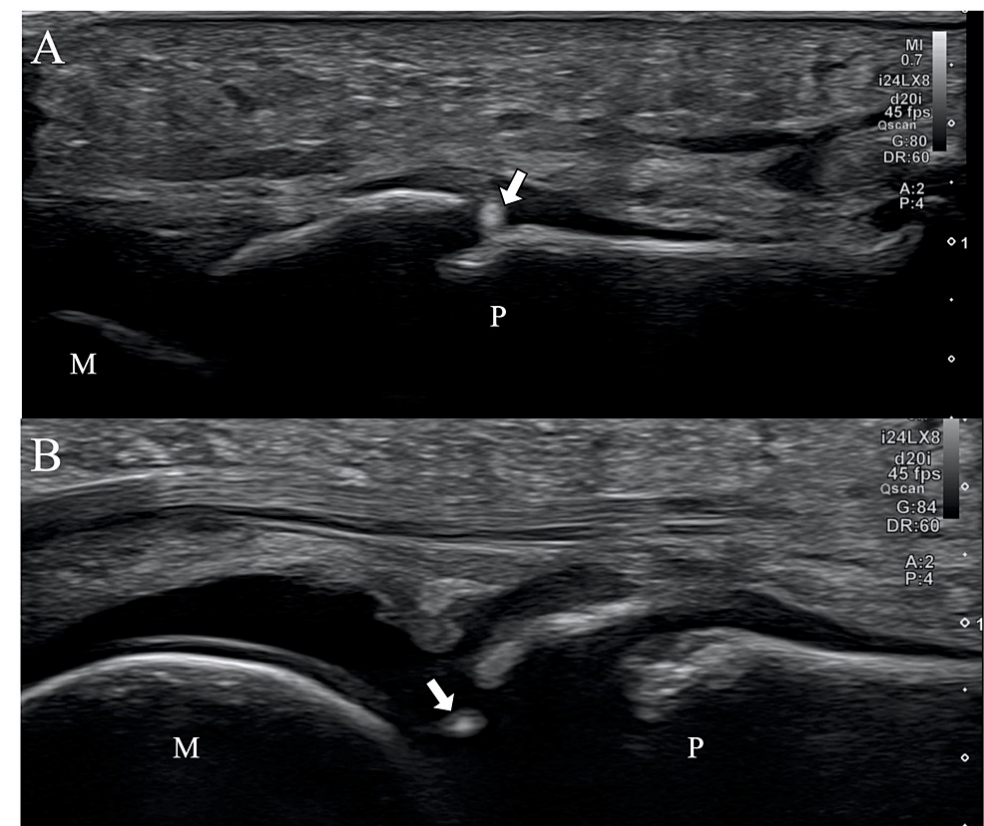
Image findings of ultrasound-guided PRP injection A: PRP was injected by inserting the needle tip into the fracture site of the proximal phalanx of the great toe. B: PRP was injected by inserting the needle tip into the metatarsophalangeal joint of the great toe. The arrow indicates the needle tip PRP: platelet-rich plasma; M: first metatarsal; P: proximal phalanx of the great toe
